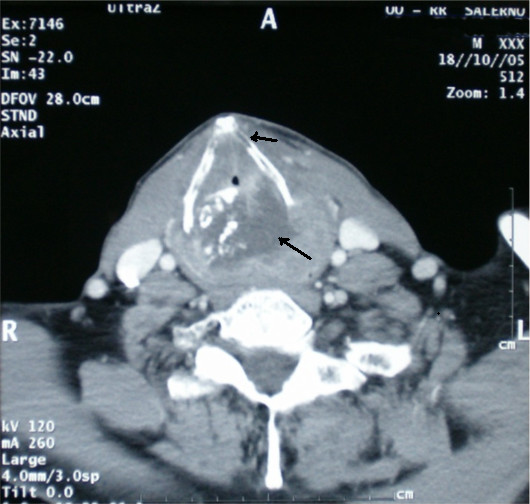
CT Scan with contrast. Tumour mass affecting glottic and supraglottic larynx, which involved the thyroid cartilage and the paralaryngeal space. In the context of the mass presence of calcification areas.
